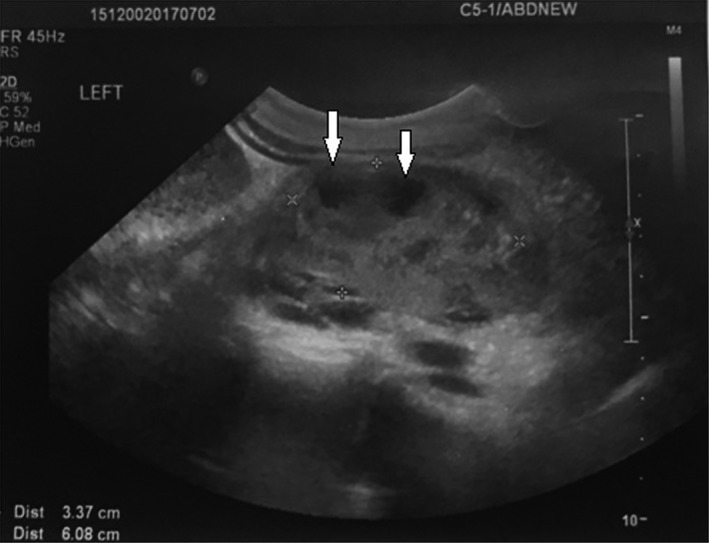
Ultrasound of the left pelvic ectopic kidney revealed multiple abscesses in its upper pole. The arrows in the figure indicate the locations of some them
